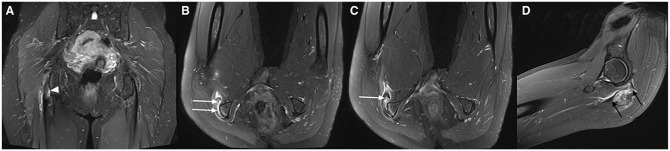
T2-weighted Dixon magnetic resonance imaging scans from a 57-year-old man with hamstring symptoms on the right side. (A) A standard coronal image shows a partial tear of the proximal semimembranosus tendon (arrowhead). (B and C) Axial images with hip flexion reveal that the tear is much larger, as almost a complete detachment of the tendon is seen (white arrows). (D) The total length of the rupture is also portrayed on a sagittal image with hip flexion (between black arrows).
